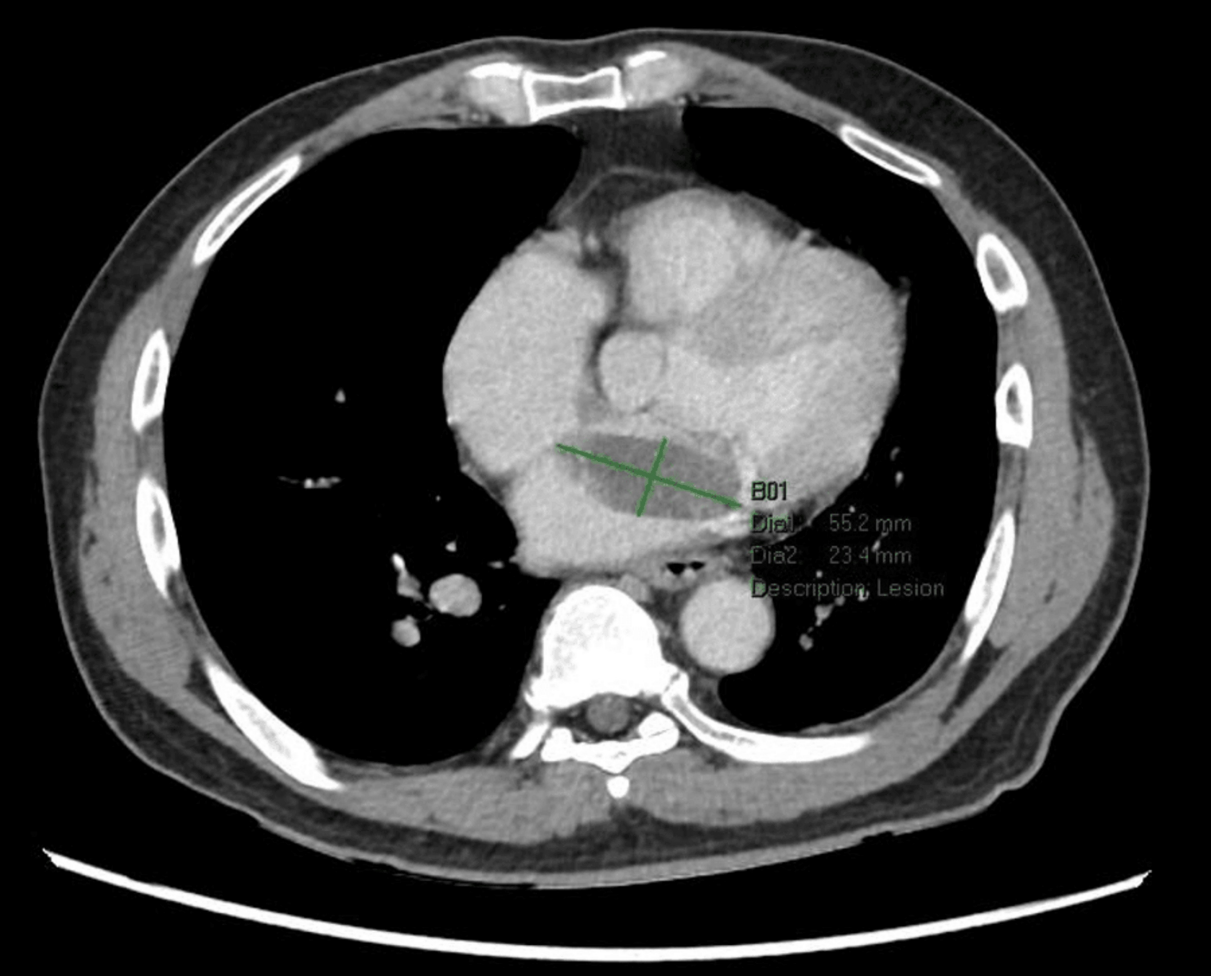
Transverse CT image of the lower thorax showing an incidental finding of a large filling defect in the left atrium with its two-dimensional measurement indicated in green.
